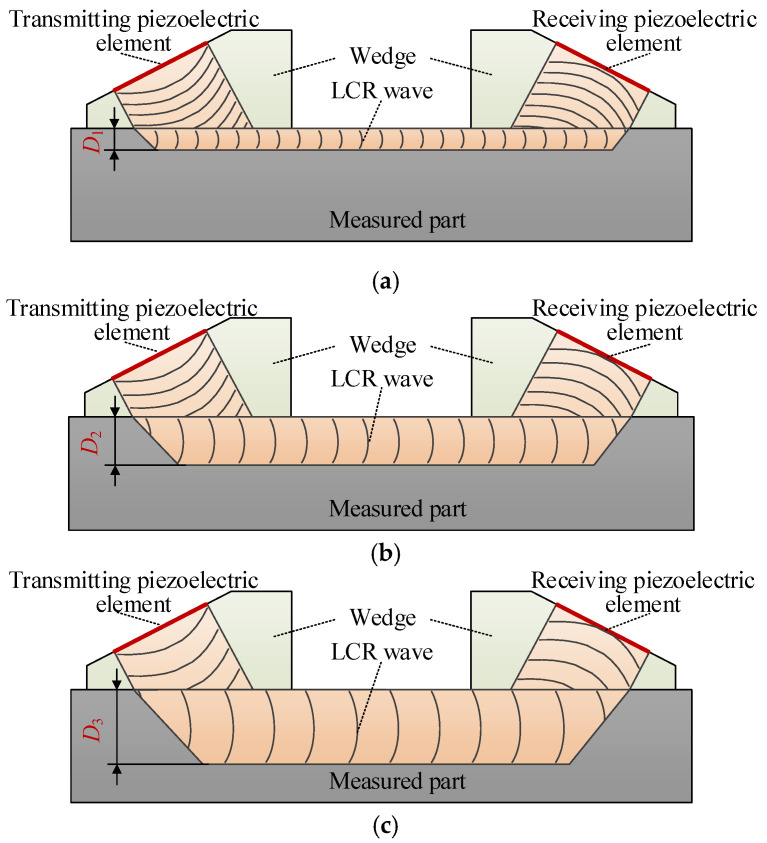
Ultrasonic transducers with different frequencies can generate LCR waves propagating at different depths in the material. (a) Propagation depth of LCR wave at *f*_1_ frequency; (b) propagation depth of LCR wave at *f*_2_ frequency; (c) propagation depth of LCR wave at *f*_3_ frequency.
